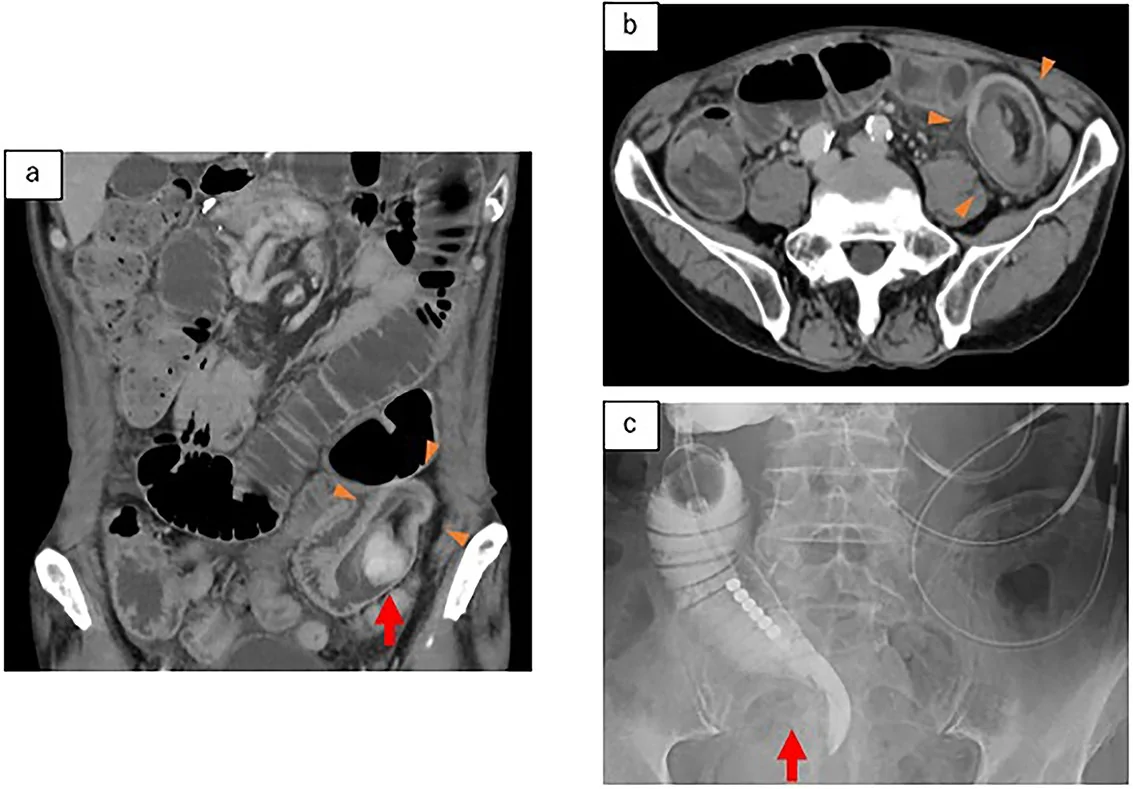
Contrast-enhanced abdominal CT images showed intussusception caused by an enhancing tumor serving as the lead point, with a target sign (arrowheads): (a) coronal view and (b) axial view. (c) A contrast study through a long intestinal tube showed a crab-claw appearance, with no distal passage of contrast medium (arrow).
